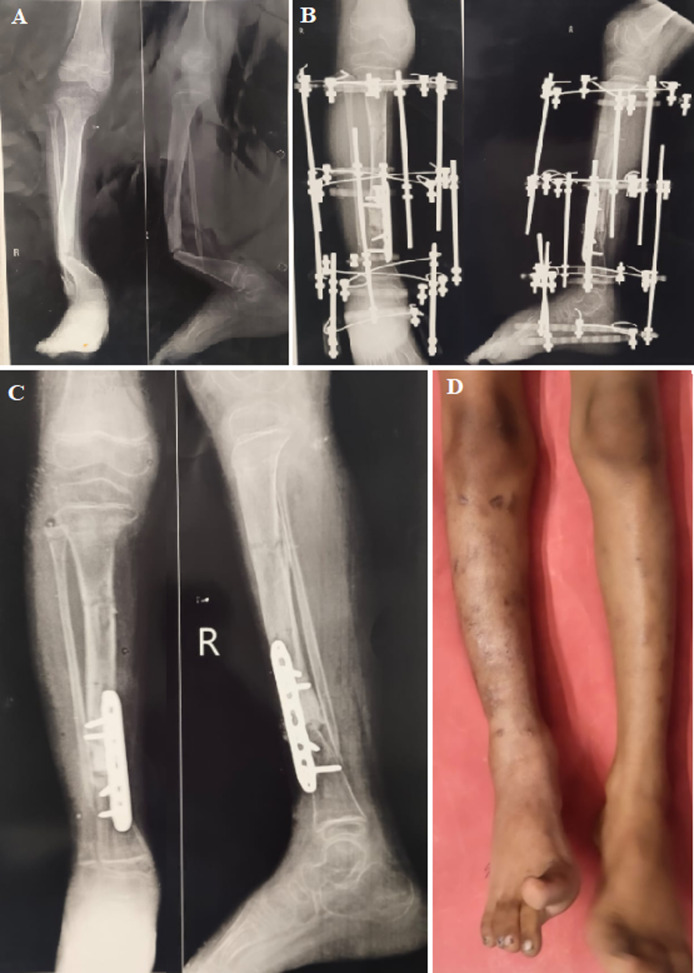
A) fracture of distal third tibia and fibula with cupping of the proximal fragment of, narrow appearance of the distal bone; B) post-operative X-ray showing fracture managed with Ilizarov ring fixator application and open reduction internal fixation with plate osteosynthesis; C) X-ray done after removal of the Ilizarov ring fixator showing union at the fracture site 2 months after the surgery; D) clinical photo of the leg showing corrected deformity
